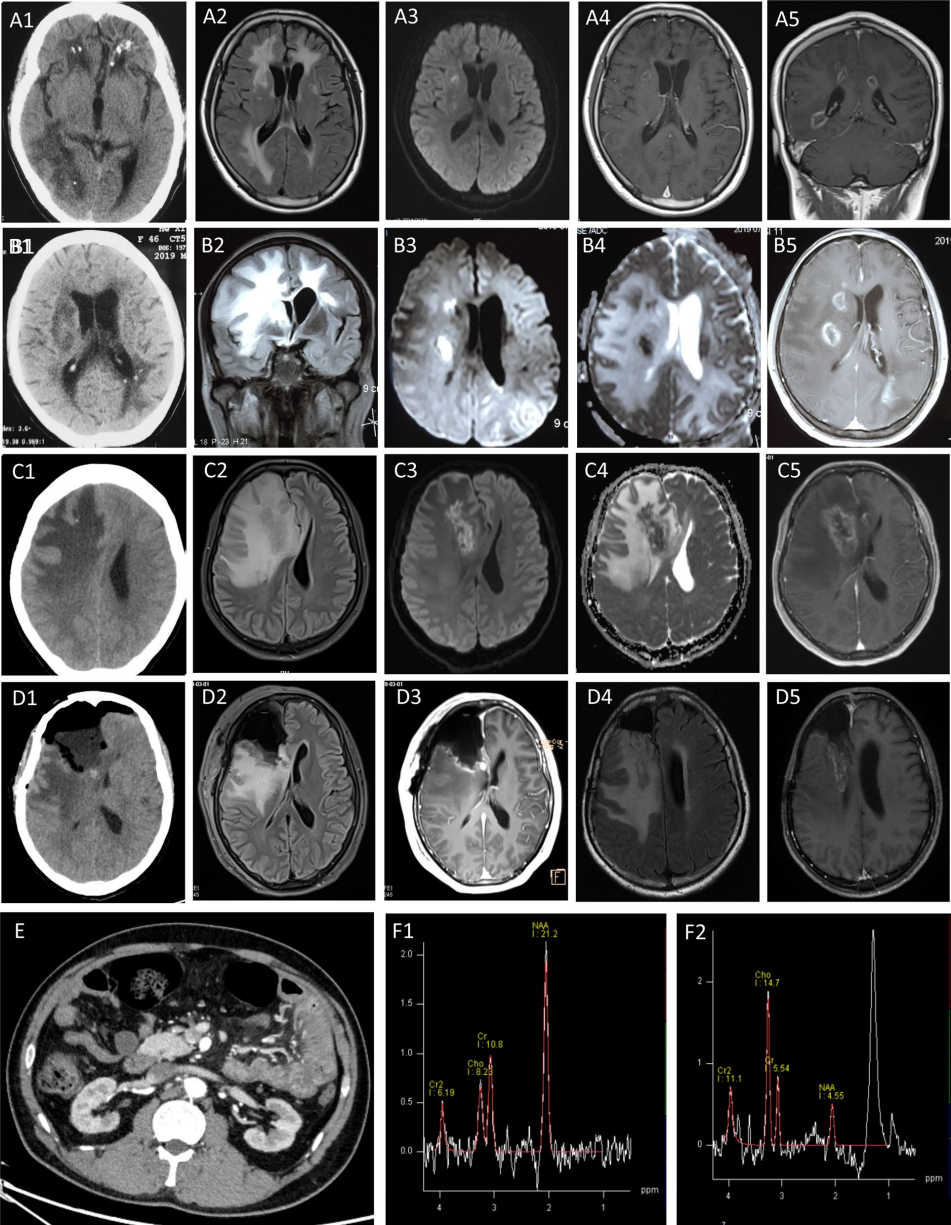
Radiological tests. a brain CT and MRI of the proband (III-7). (A1) Non-contrast CT scan showed extensively hypodense lesions with focal calcifications. (A2) FLAIR images showed diffuse leukoencephalopathy bilaterally. (A3) DWI indicated central restricted diffusion. (A4, A5) Post-contrast MRI revealed multiple irregular rim-enhancing lesions. b brain CT and MRI of patient 2 (III-1). (B1) Non-contrast CT scan showed extensively hypodense lesions with focal calcifications. (B2) Coronary FLAIR images showed diffuse leukoencephalopathy. (B3, B4) DWI paired with ADC indicated central restricted diffusion. (B5) Post-contrast MRI showed multiple irregular rim-enhancing lesions in the periventricular region. c Preoperative neuroimages of patient 3 (III-4). (C1) Non-contrast CT scan showed irregular hypodense lesions in the right frontal lobe and basal ganglia region with mass effect and a tiny calcified dot. (C2) FLAIR images showed iso- to hyperintense lesions with peripheral edema. (C3, C4) DWI paired with ADC indicated central restricted diffusion. (C5) Post-contrast MRI showed a garland-like enhancement pattern. d Postoperative neuroimages of patient 3 (III-4). (D1) Non-contrast CT scan showed postoperative changes in the residual cavity. (D2, D3) FLAIR and enhanced MRI done immediately after the surgery showed removal of the lesion and enhancement of the surrounding meninges. (D4, D5) One month later, a follow-up MRI showed newly occurred rim-enhancing lesions posterior to the resected region, with residual cavity shrank and meningeal enhancement diminished, which was misdiagnosed as radiation-induced necrosis by the radiologist. e Abdominal CT of the proband (III-7). Post-contrast CT showed segmental intestinal wall edema in the mid-upper abdomen and mesenteric inflammatory changes (edema, panniculitis, increased blood vessel density, and vessel dilatation). f MRS pattern. (F1) normal tissue. (F2) a ring-enhancing lesion of patient 3 (III-4)
